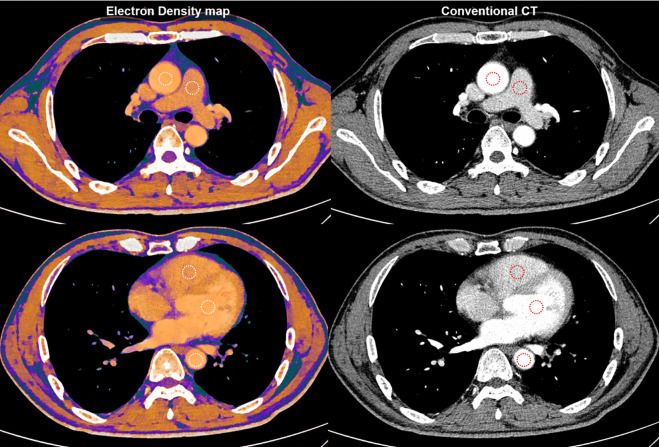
ROI placement on conventional CT and ED map. ROIs are drawn on the ascending aorta and pulmonary trunk at the level of the pulmonary trunk, and on the right ventricle, left ventricle, and descending aorta at the level of the left ventricle.
